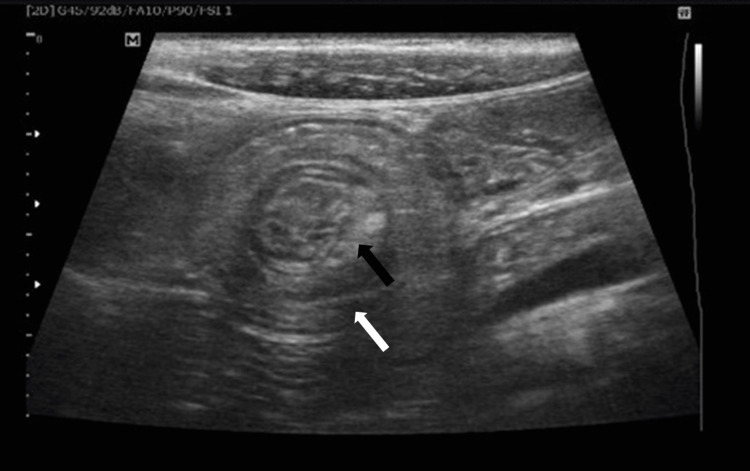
High-resolution transverse sonography of the abdomen via a linear transducer placed in the right iliac fossa region The image shows a swirled pattern of alternating hyperechogenicity and hypoechogenicity giving the classical "target" appearance representing the alternating layers of mucosa, muscularis, and serosa. Findings are consistent with intussusception with the inner bowel (black arrow) representing the intussuscipiens and the outer bowel (white arrow) representing the intussusceptum
